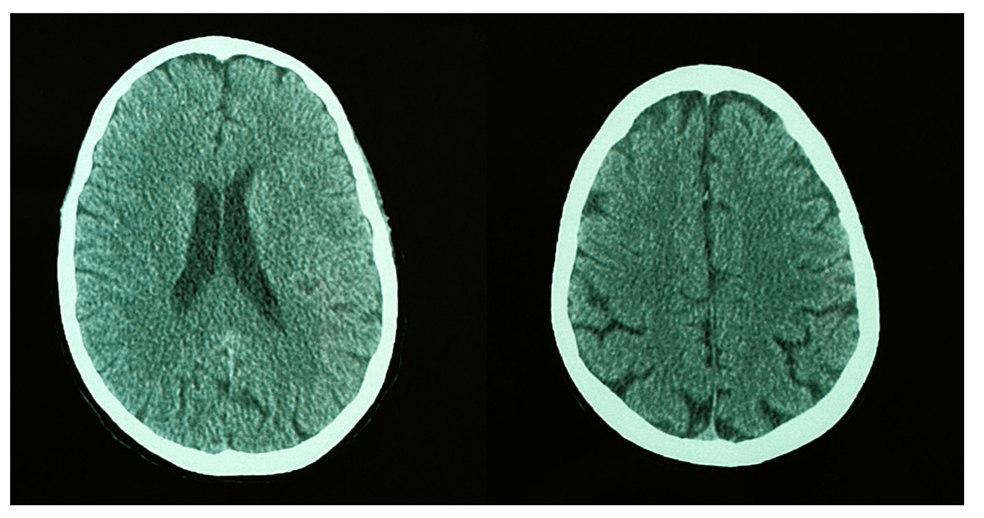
Normal computed tomography (CT) scan in axial cerebral reconstruction
